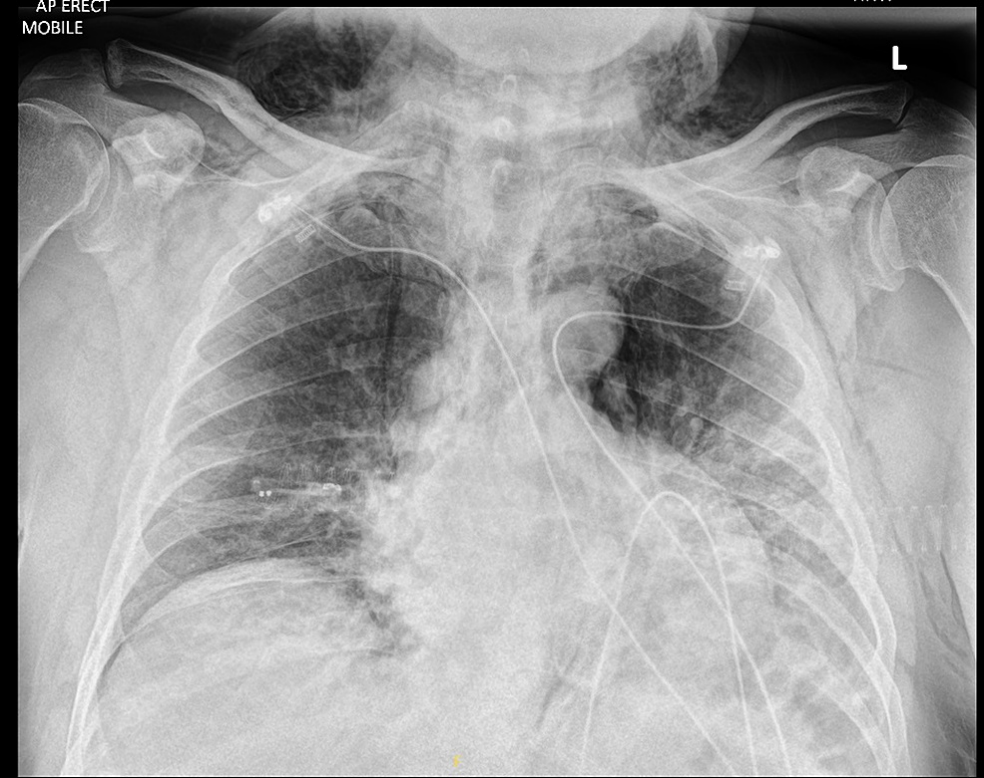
Repeat chest X-ray following CTPA demonstrating the progression of viral pneumonitis and surgical emphysema CTPA: computed tomography pulmonary angiogram
